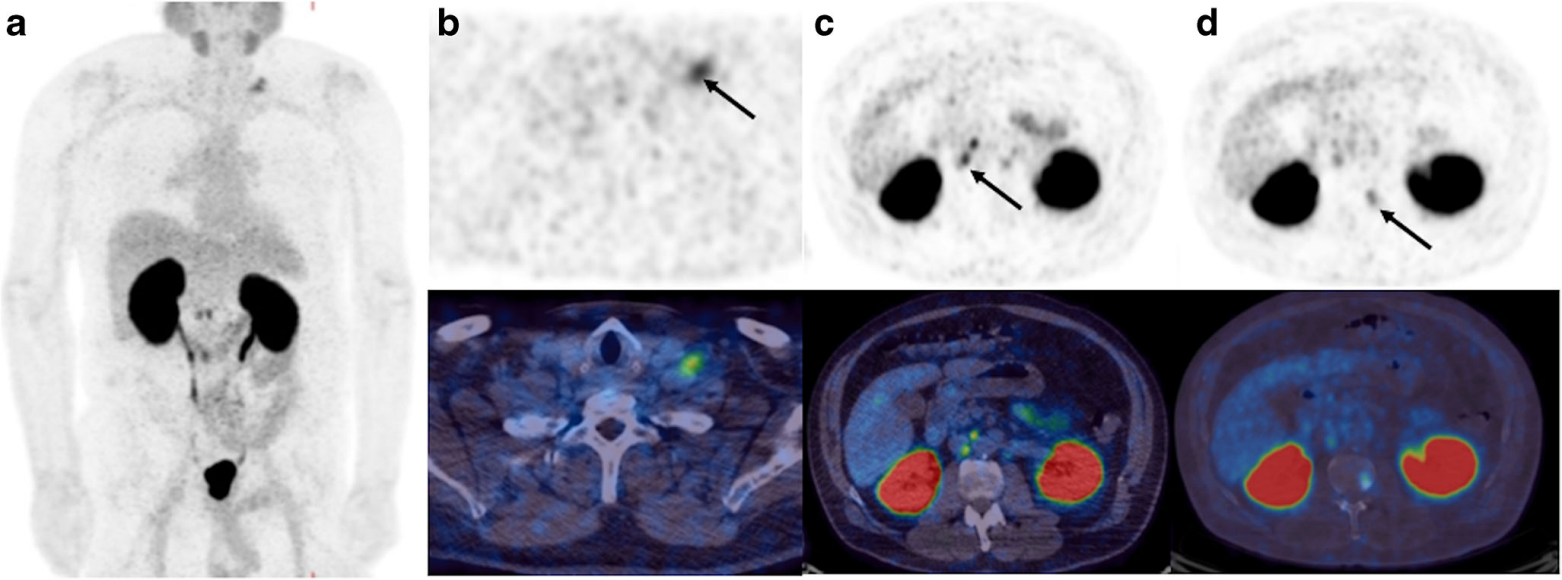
^68^Ga-THP-PSMA PET-CT scan of a man with a rising PSA (3 μg/L) after a previous radical prostatectomy. a Maximum intensity projection image. b There is evidence of nodal disease in the left supraclavicular fossa (arrow) and c retroperitoneum (arrow) as well as d a vertebral bone metastasis (arrow)
